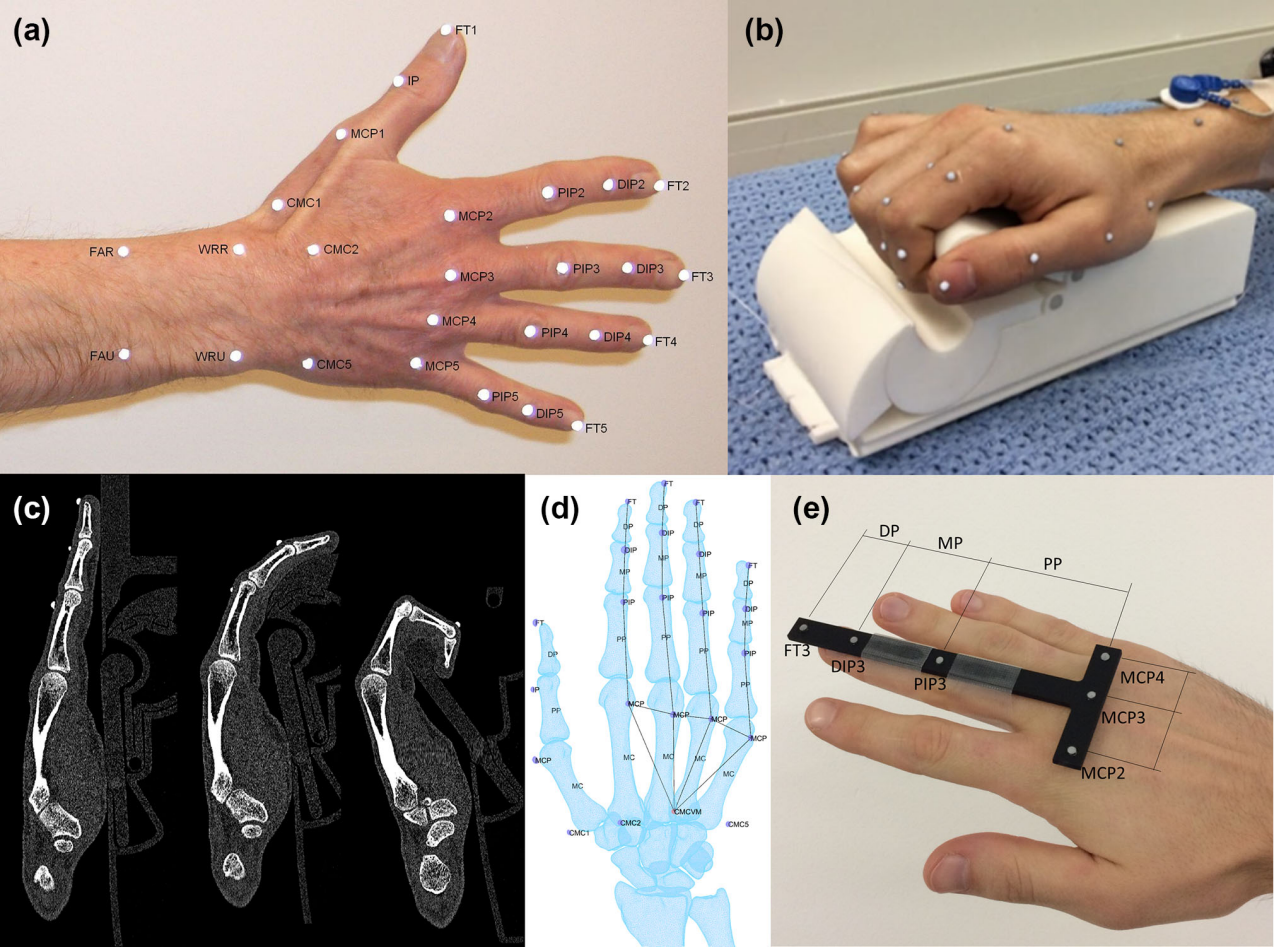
(a) HAWK 26-marker motion capture set, (b) finger pose support jig and (c) exemplar CT sections in three scanned positions. Note that markers are visible in CT data. (d) extracted bone and marker meshes, showing distal- and palmar-direction movement of the PIP marker relative to the reference proximal phalanx bone as joint flexion increases. (e) wand for system noise measurement, indicating ‘joint’ markers and finger segments.
